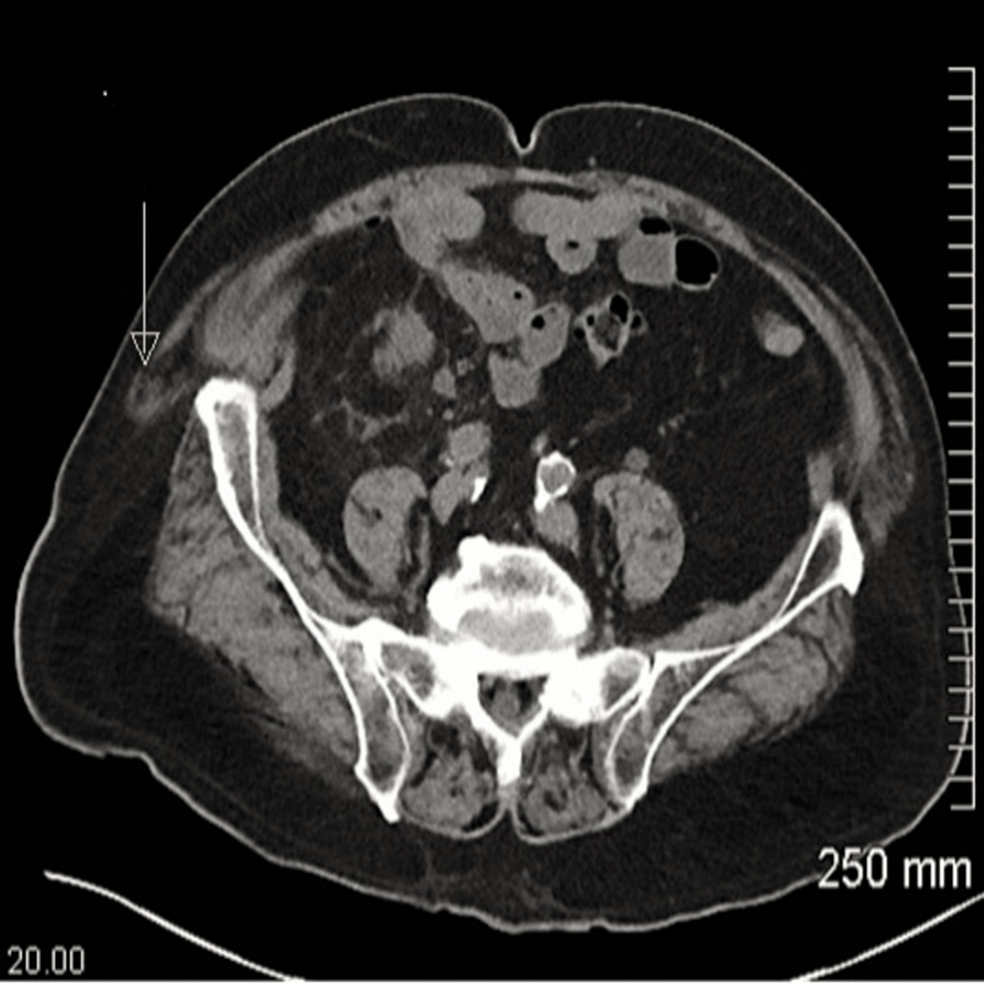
Computed tomography urogram without contrast with arrows pointing to the right distal ureter being compressed by the hernia sac
